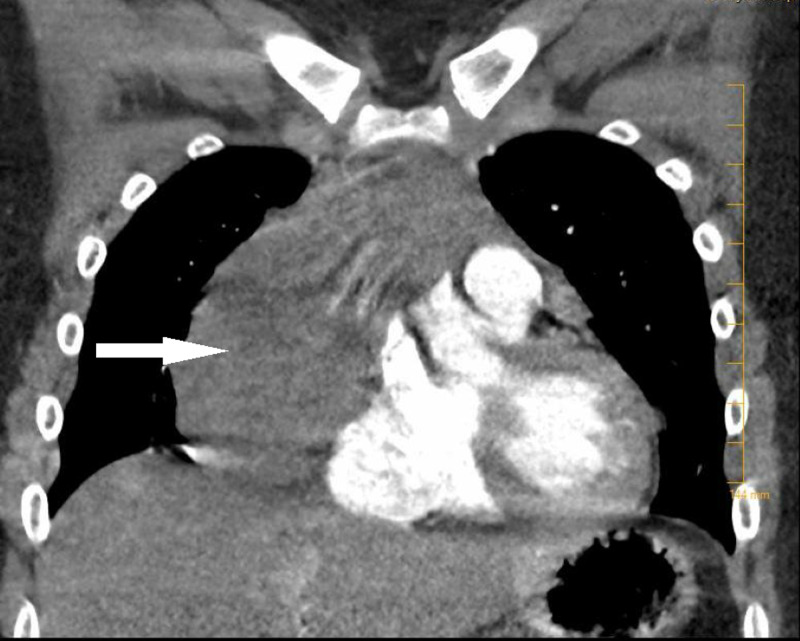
Coronal view computed tomography of chest scan with contrast 10.7 cm craniocaudal x 4 cm anteroposterior x 10.1 cm transverse mass (white arrow) inseparable from the right heart border pericardium, displacing the heart to the left with associated compression of the superior right atrium and the superior vena cava
